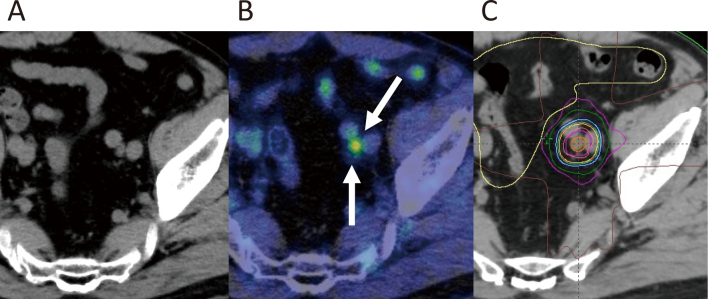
A male patient with prostate cancer underwent salvage radiation therapy for biochemical recurrence after surgical resection. However, his prostate-specific antigen levels gradually increased after five years. Computed tomography (CT) could not detect any recurrent lesions (A); however, ^18^F- fluorodeoxyglucose positron emission tomography combined with CT clearly revealed suspected lymph node disease (B, indicated by white arrows). This lesion was treated using stereotactic radiation therapy using a total dose of 50 Gy in 10 fractions. Dose distributions of the treatments (C). Prostate-specific antigen levels dramatically decreased after treatment.
